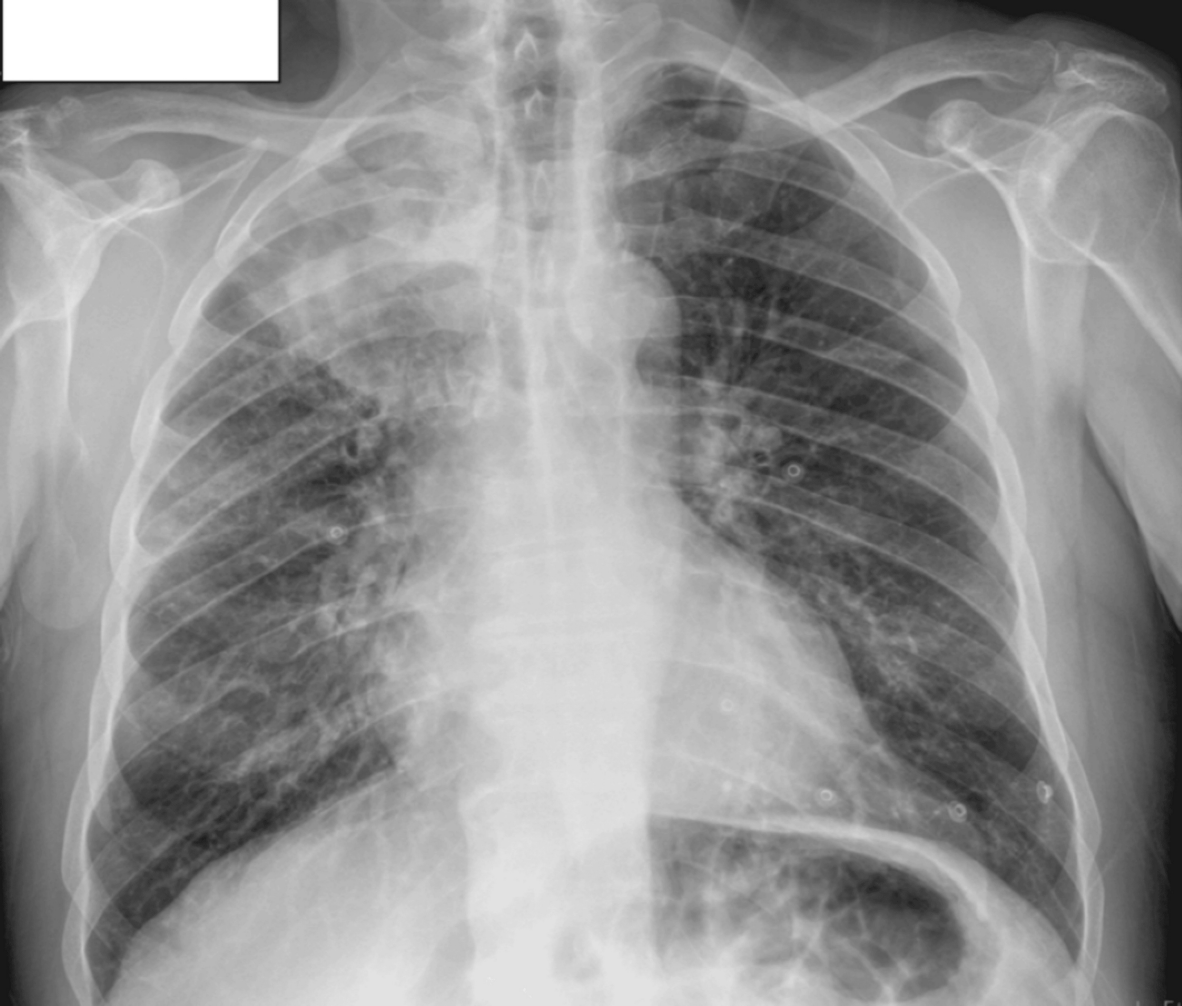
Initial Chest X-ray at Presentation
